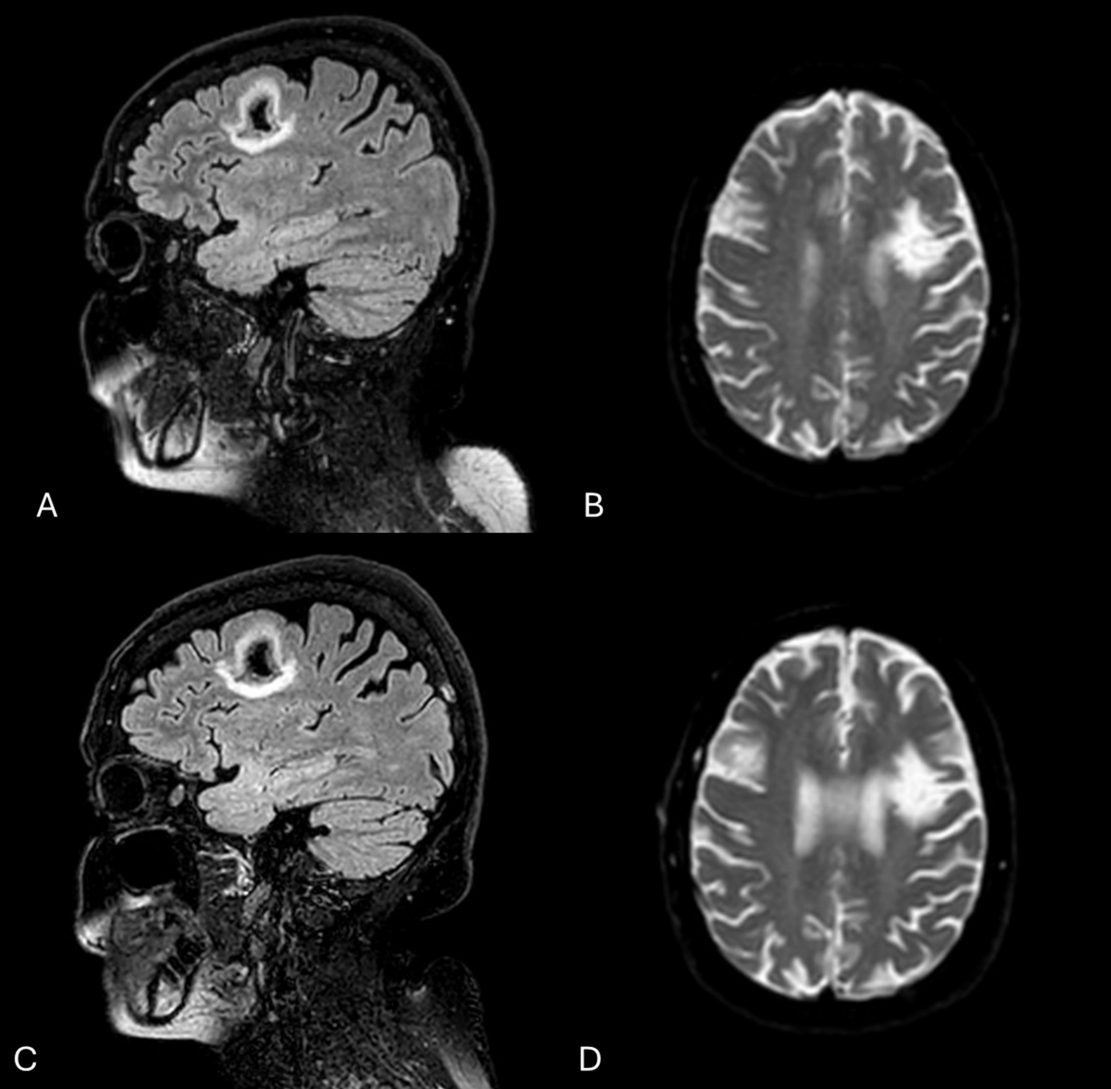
Brain MRI performed in 2014 showing a resolved left parietal infarct on FLAIR (A) and DWI (B) sequences. Brain MRI images, FLAIR (C) and DWI (D), obtained in 2022, were unchanged.
